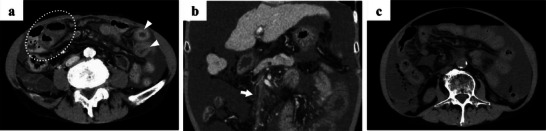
(a) The arterial phase of contrast‐enhanced computed tomography (CT) image demonstrates the profound contrast difference between the normal (arrowhead) and hypoenhancing ischemic (dotted line) loops. (b) Venous phase contrast‐enhanced CT reveals extensive mesenteric venous thrombosis (arrow) with a large volume of ascites. (c) CT demonstrates intraperitoneal free air, although the perforation site was not clearly identified.
